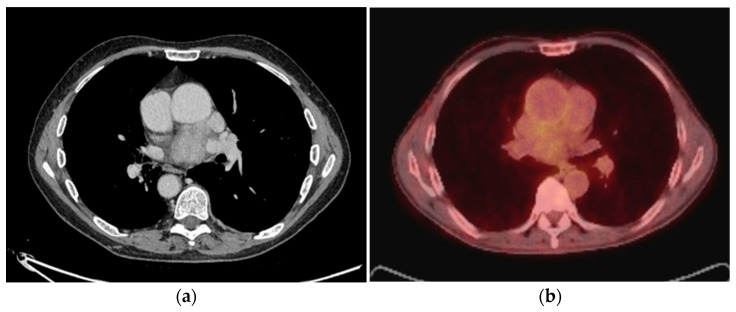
Biopsy-proven Mediastinal EP, case from our institution (a) Axial CT image shows an enhancing soft tissue lesion in the subcarinal space; (b) PET-CT image at the same level shows the mediastinal mass with mild heterogenous avidity.
